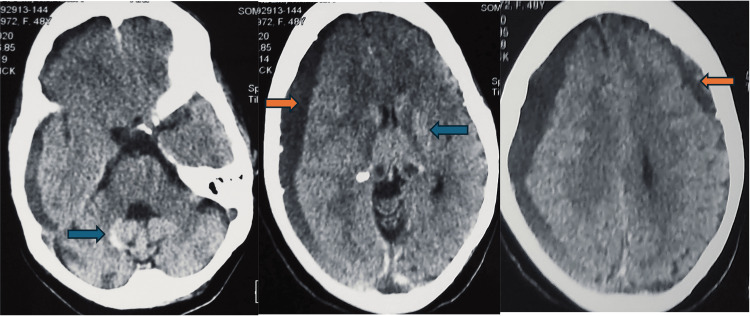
Axial non-contrast brain CT scan showing a right fronto-temporo-parieto-occipital subdural hematoma and a left frontoparietal subdural hematoma (orange arrows) with abnormal high-density areas in the basal ganglia (blue arrows)
